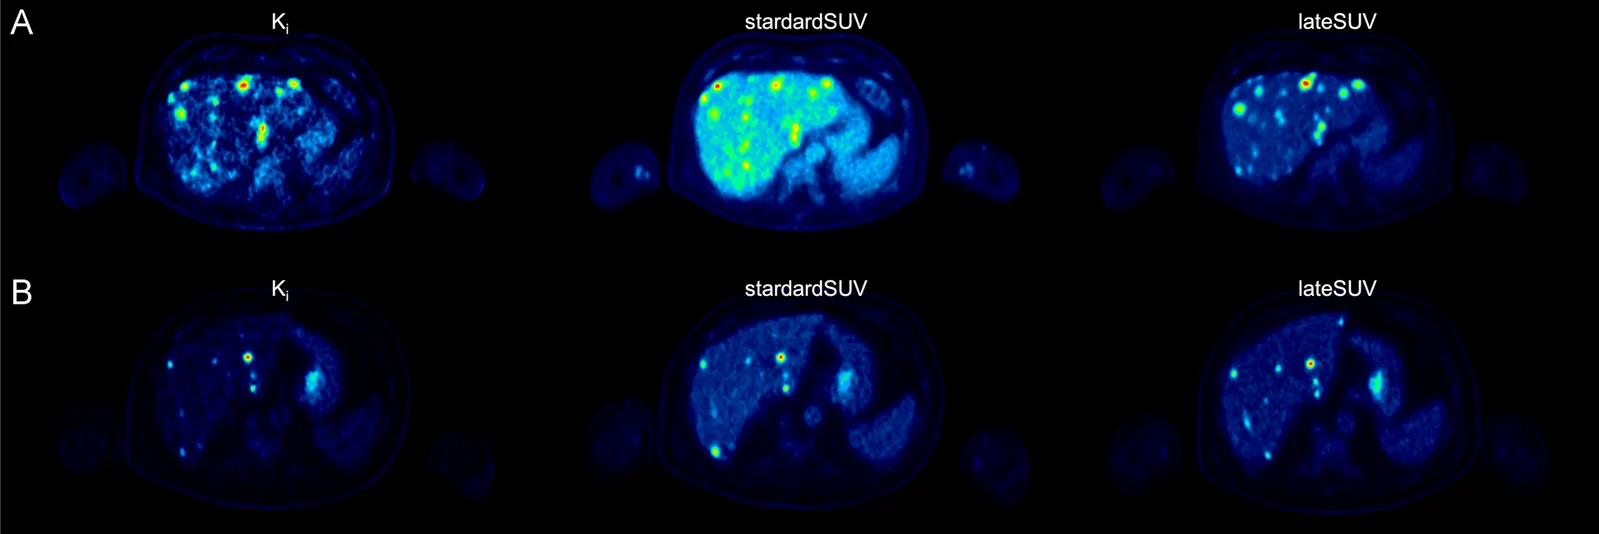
In patient No. 22 (row A), lesion conspicuity was greater on late SUV images compared with K_i_ images, with moreover, more lesions detected and clearly improved delineation relative to standard SUV images. In patient No. 7 (row B), a higher number of lesions was detected on late SUV images compared with both K_i_ Patlak and standard SUV images
